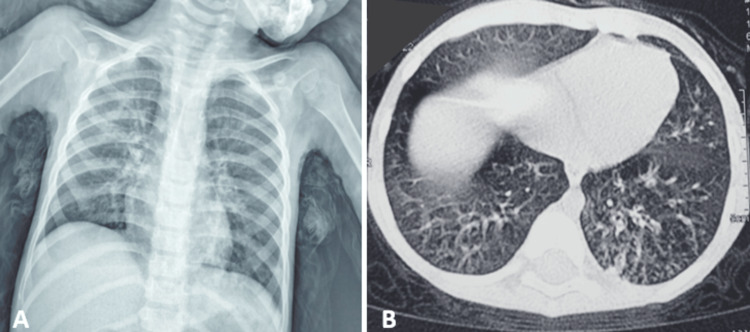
Image A: The frontal chest X-ray reveals a bilateral alveolo-interstitial syndrome with a predominance on the right side. Image B: The thoracic CT scan without contrast injection, in axial sections and parenchymal window, reveals bilateral lung involvement predominantly at the right base.
